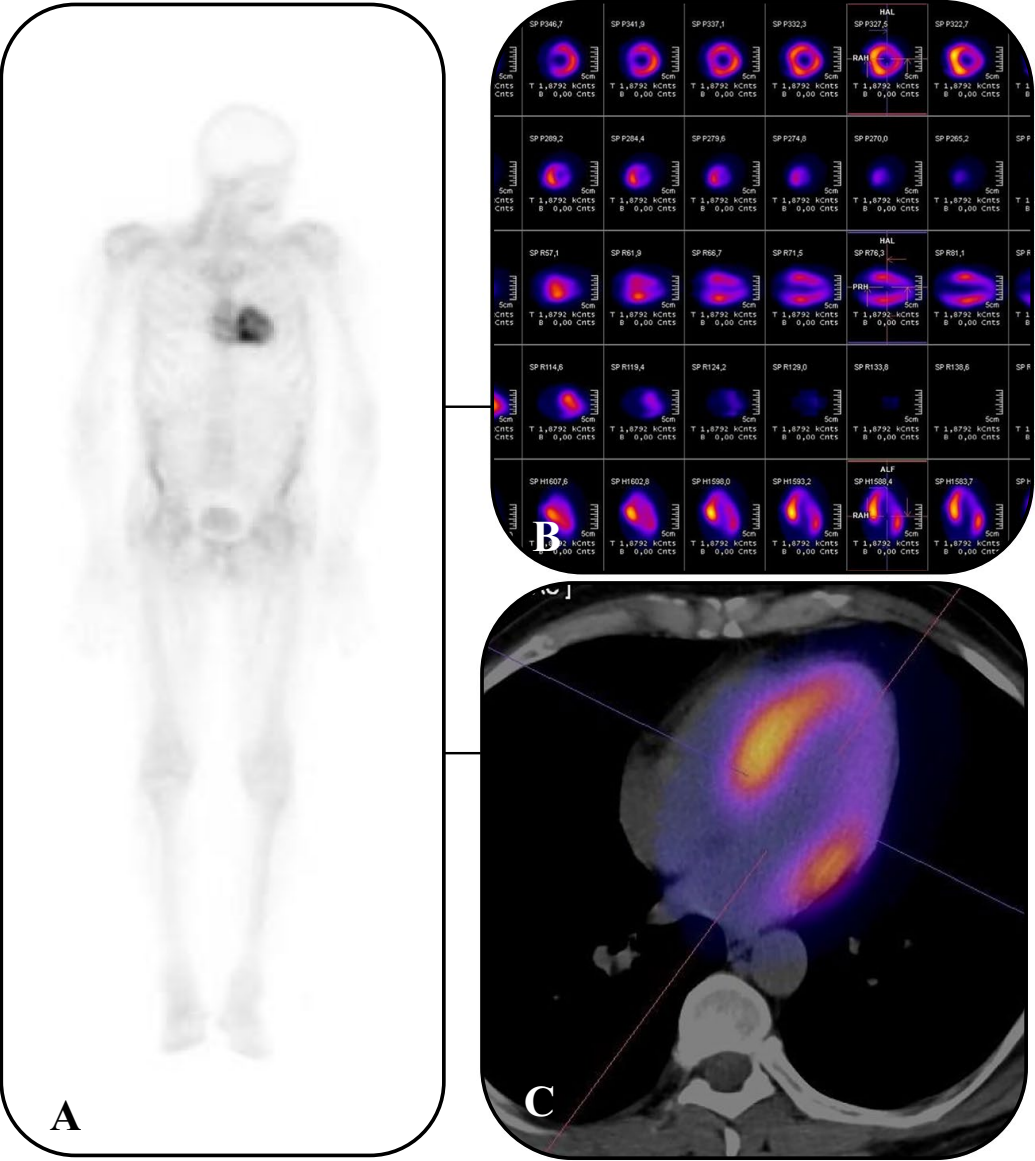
Imaging results in an asymptomatic first-degree relative of a patient with hereditary cardiac transthyretin amyloidosis are consistent with advanced cardiac involvement. A—planar whole-body scintigraphy with [99mTc] Tc-DPD (grade 3). B—SPECT imaging with [99mTc]Tc-DPD (after attenuation correction). C – hybrid SPECT/CT imaging. CT – computed tomography; DPD—3,3-disphono-1,2-propanodicarboxylic acid; SPECT—single-photon emission computed tomography
